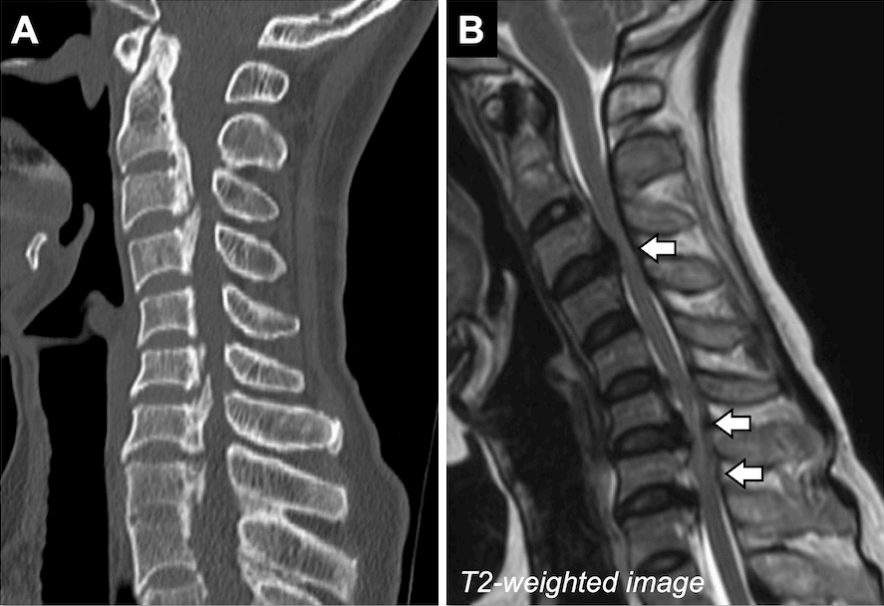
A) Spine computed tomography showed ossification of the posterior longitudinal ligament from C2 to T1. B) Magnetic resonance imaging of the cervical spine revealed intramedullary high signal intensity on T2-weighted images at the C3/4 and C6-T1 levels (arrows).
